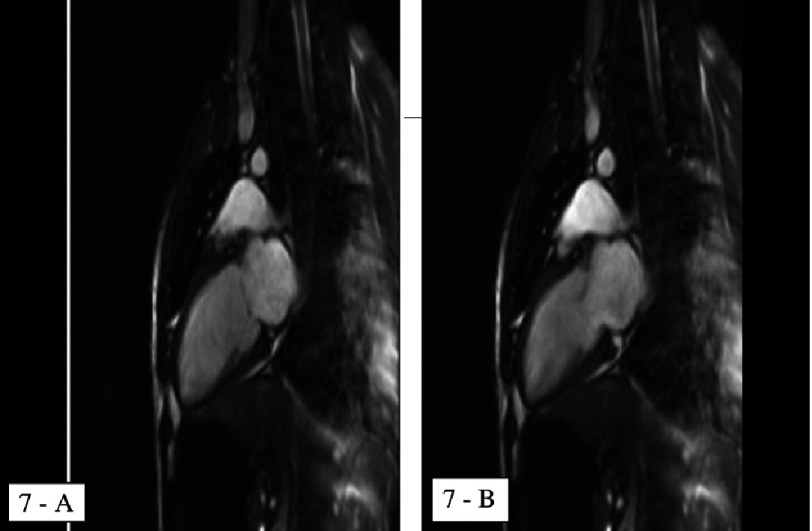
Cardiac MRI showing global LV hypokinesia. 7a: 2-chamber view end-diastolic, 7b: 2-chamber view end-systolic.
